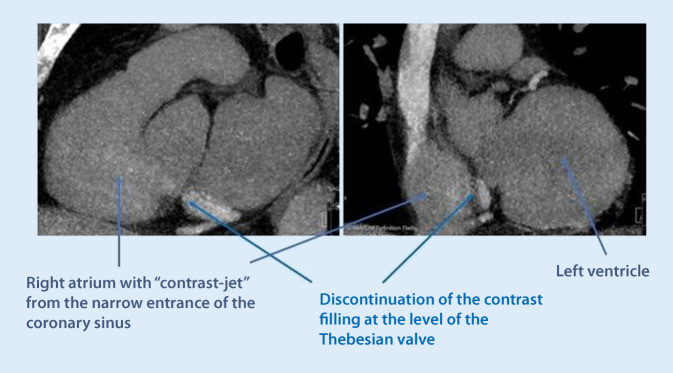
Cardiac computed tomography showing the right atrium and ostium of the coronary sinus. There is contrast passage from the coronary sinus (CS) to the atrium, but the prominent Thebesian valve appears as a discontinuation of the CS right at the level of the ostiumiv.Anatomical variants may affect the success rate of CS cannulation and lead placement. Narrowing of the CS ostium is not an uncommon finding and, in some cases, makes it impossible to pass with a sheath. This often occurs secondary to previous lead placements or other device placement in the CS, but can also occur without prior interventions. The problem can be overcome by either progressive balloon dilatation with a percutaneous coronary intervention (PCI) technique, or by using a stable steerable sheath that is positioned immediately outside the ostium, providing enough support for a sub-select catheter to enter. One alternative is to use a large 9‑F inner-diameter sheath outside the ostium and place a superstiff 0.035 in wire deep into the CS to keep the sheath stabilized and in place. Assistance in the CS cannulation via simultaneous femoral access with a diagnostic EP catheter may also increase the success rate of CS cannulation and lead placement in these difficult cases (Fig. 7).
